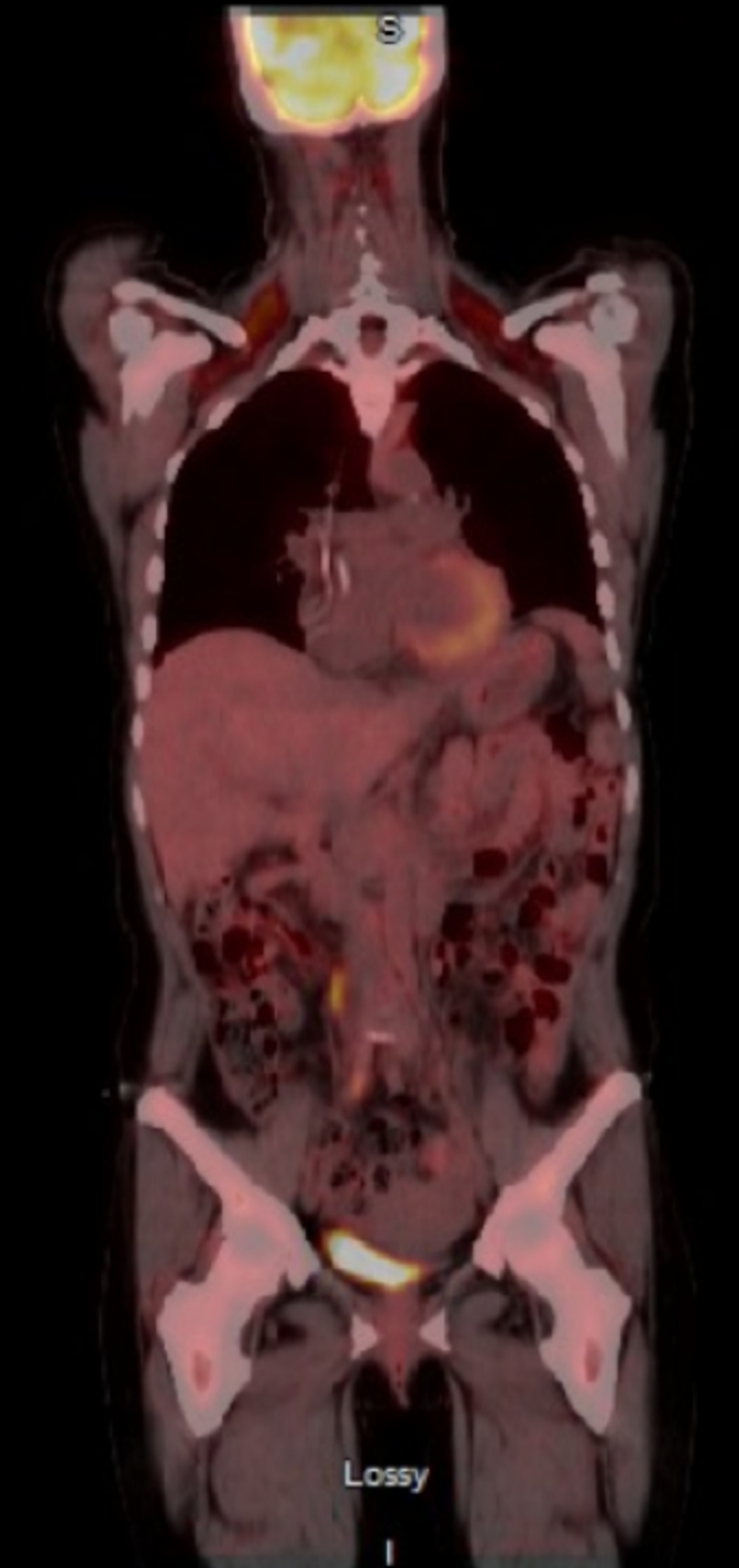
Post-treatment positron emission tomography/computed tomography (PET/CT) No fluorodeoxyglucose (FDG) uptake noted.
